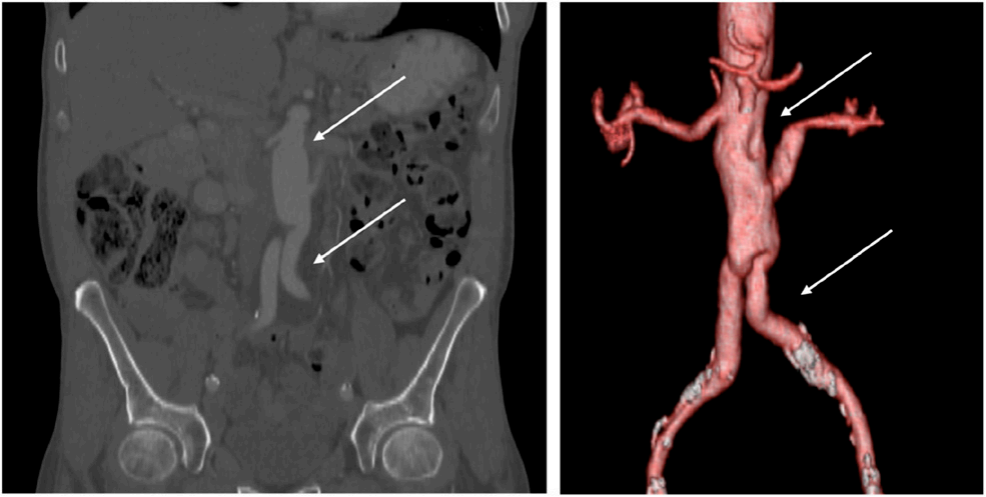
Control CT angiography and reconstruction of the chest, abdomen, and pelvis, which shows the vascular prosthesis. The proximal prosthesis end is sutured below the right renal artery, and both distal ends of the bifurcation to common iliac arteries (white arrows).
